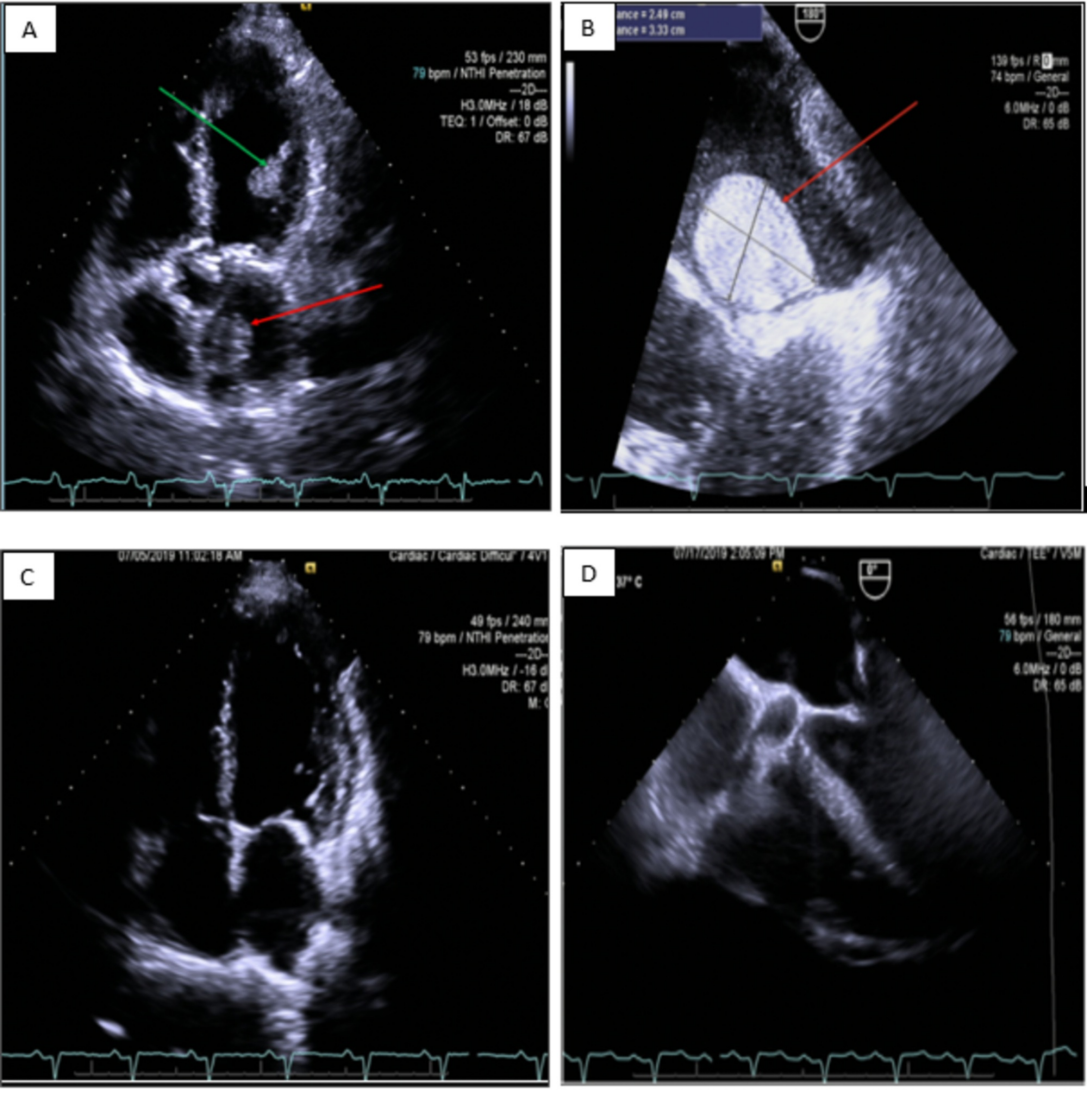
A: Pre-anticoagulation TTE. LA mass suggestive of myxoma (red arrow) as well as a LV thrombus (green arrow). B. Pre-anticoagulation TEE: 2.49 cm x 3.33 cm mass (red arrow) suggestive of myxoma on anteromedial aspect of LA. C: Post-warfarin TTE three months later. Thrombi in the LV and LA have resolved. D. Post-warfarin TEE three months later. No evidence of myxoma. TTE: transthoracic echocardiogram; TEE: transesophageal echocardiogram; LA: left atrium; LV: left ventricle.
